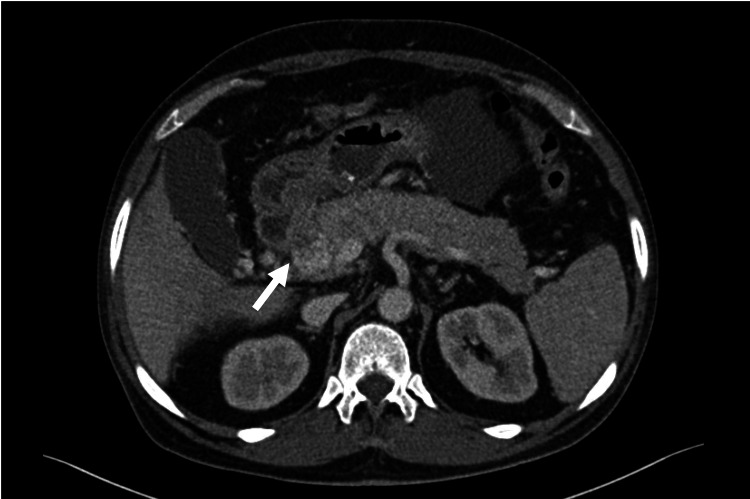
Abdominopelvic CT Abdominopelvic CT (axial). The image shows the obliteration of the fat plane between the pancreatic head and the duodenal sweep (arrow) with associated peripancreatic lymphadenopathy CT: computed tomography
